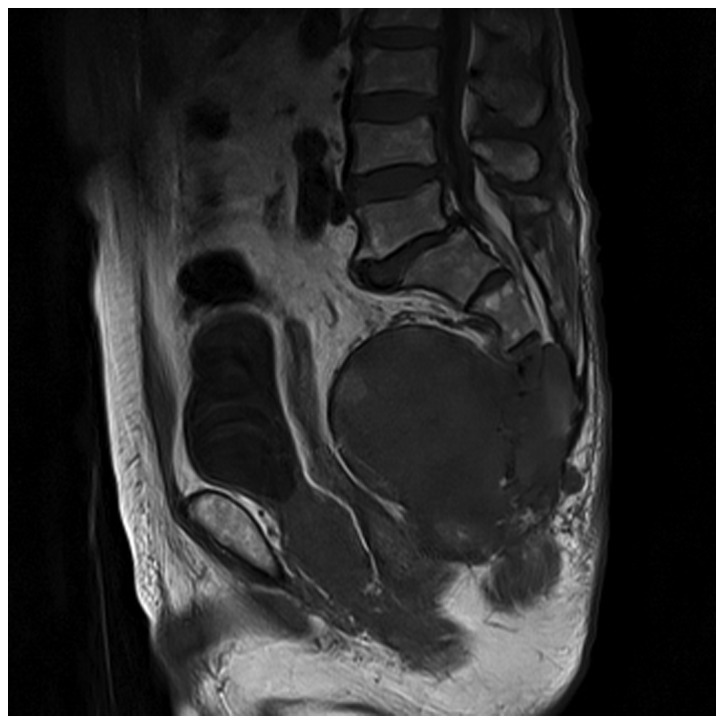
Magnetic resonance image demonstrated a large sacral tumor that spread into the soft tissue and compressed the rectum.
